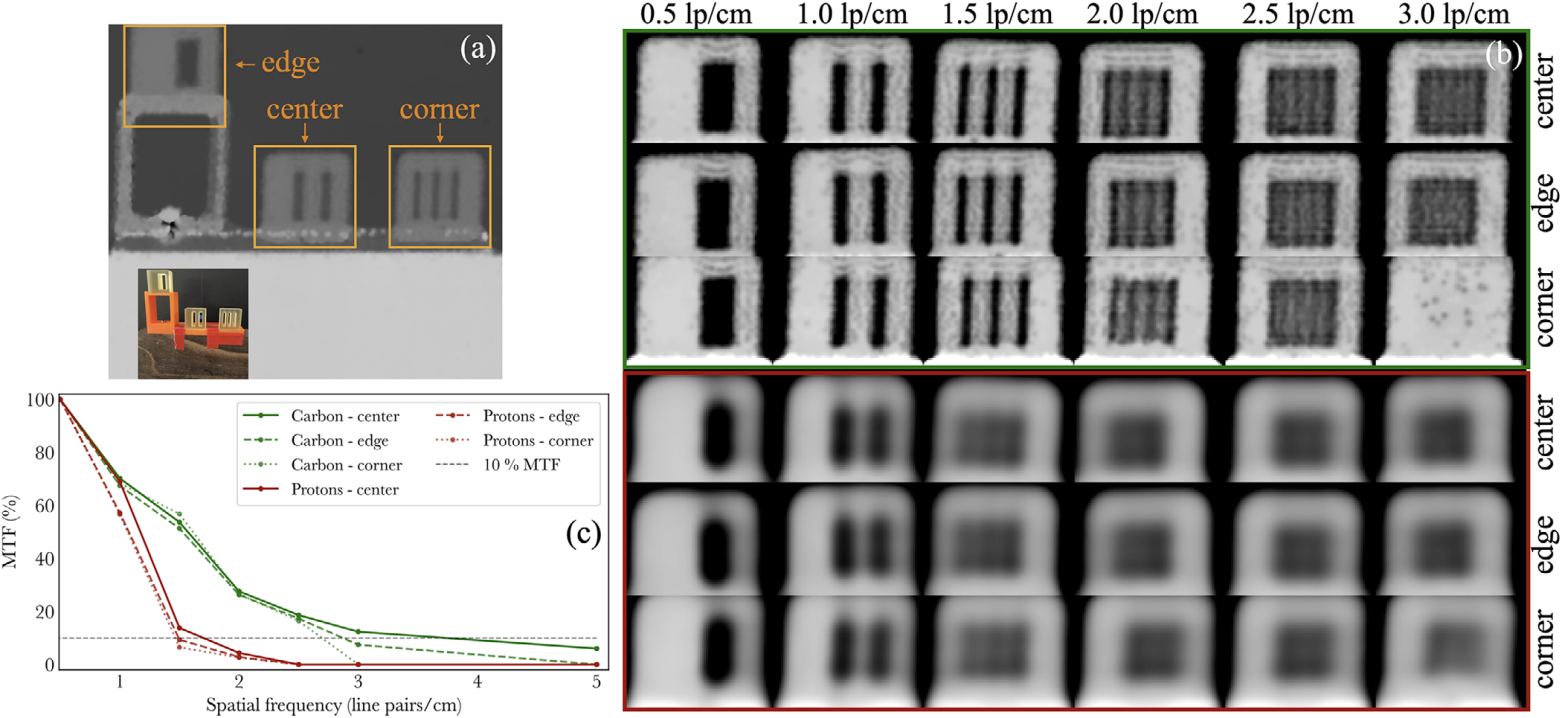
Evaluation of the spatial resolution for pRads and cRads for different locations in the imaging FOV. The locations are depicted in (a), while radiographs of each lp module are reported in (b). The corresponding modulation transfer functions associated with the modules of (b) are shown in (c). The dynamic range of pRads in (b) is from 10 to 12 cm. cRads, carbon ion radiographs; FOV, field of view; lp, line pair; pRads, proton radiographs.
